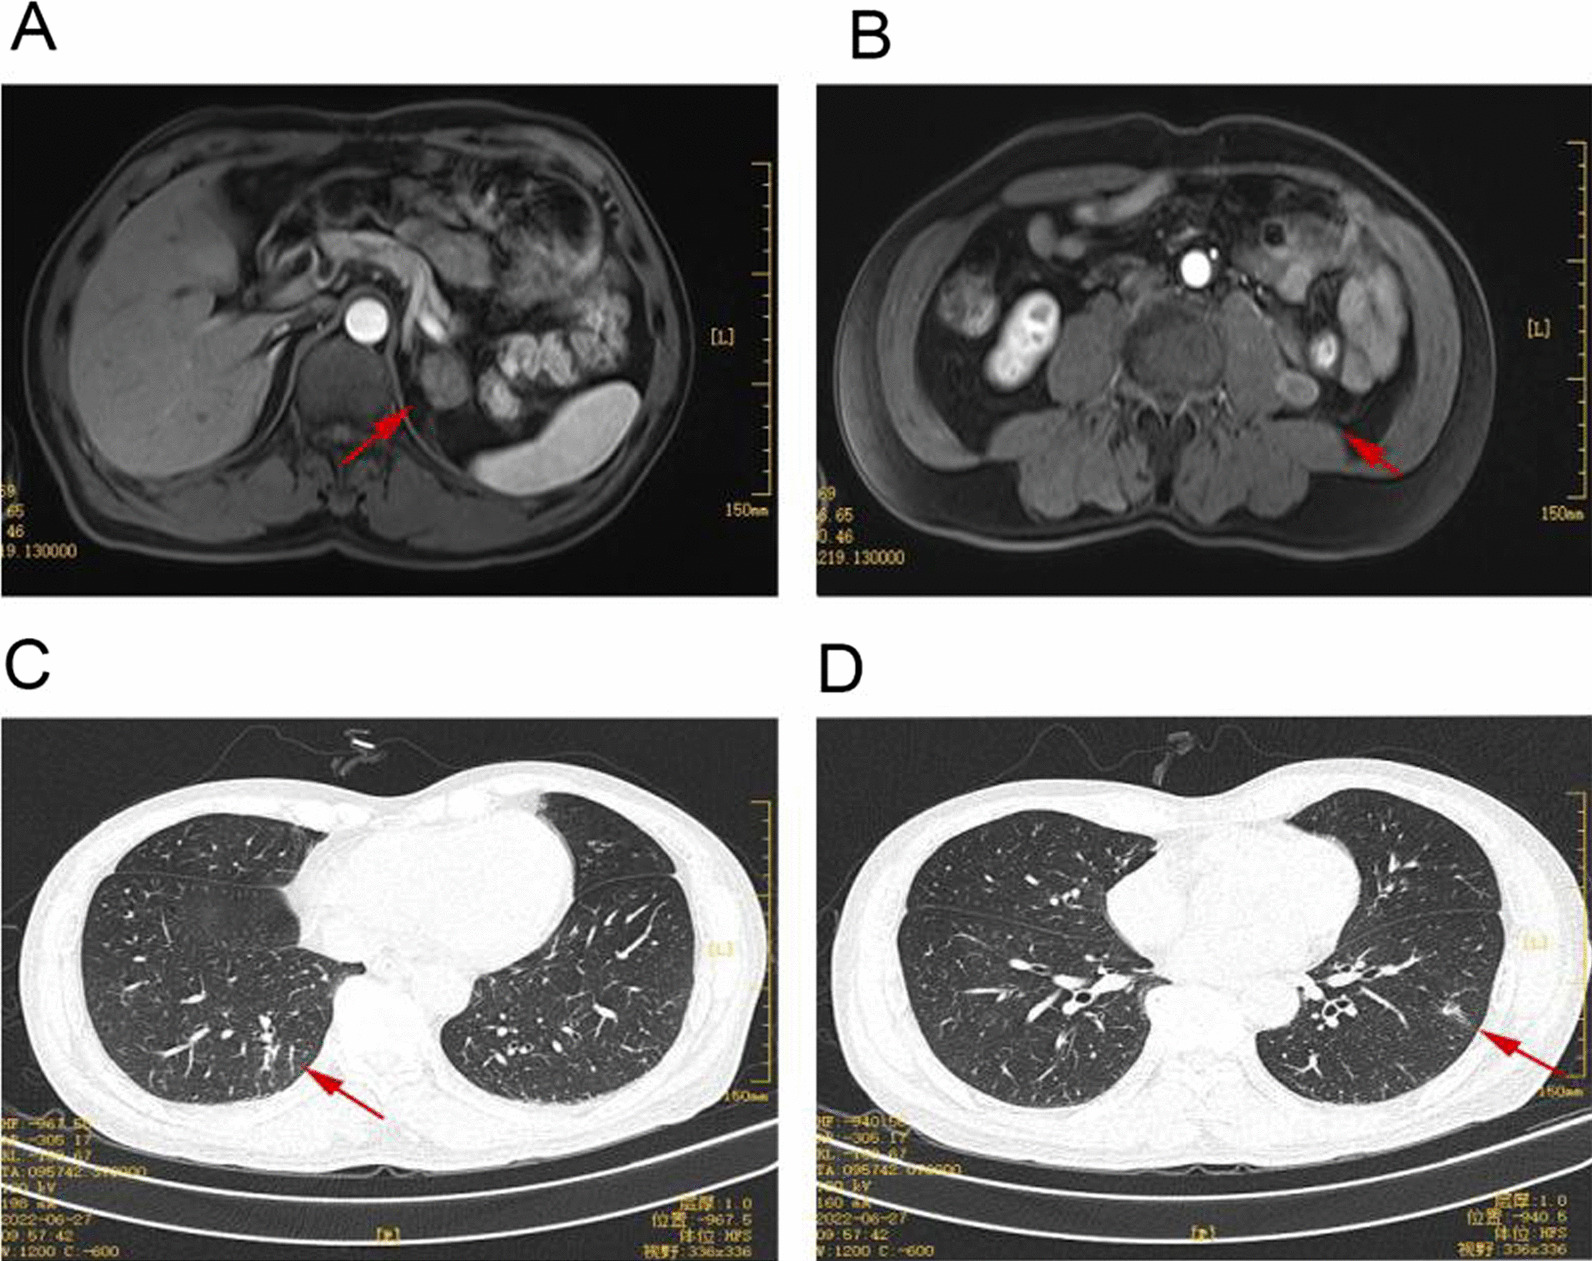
A, B Abdominal MRI images show the tumor in the left adrenal region and near the left psoas muscle with the same sizes. C, D The chest CT images show smaller shadows of multiple nodules in both lungs than before
